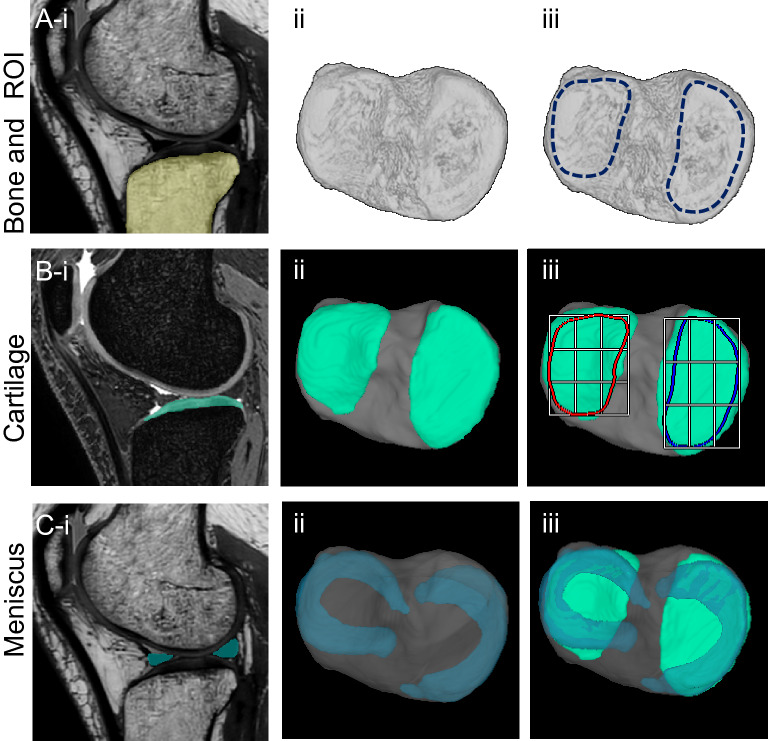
3D MRI analysis of the tibial surface of a representative control knee. (A) Bone and ROIs. The PDW images were used for automatic segmentation of the bone region (yellow) (i), the 3D image was then reconstructed (ii), and the medial and lateral tibial articular surfaces were defined as the ROIs (iii). (In this image, the ROIs were drawn manually for explanatory purposes.) (B) Cartilage. The SPGR images were used for automatic segmentation of the cartilage region (green) (i), the tibial articular cartilage and bone were reconstructed in 3D (ii), and ROIs with nine subregions were overlapped (iii). (C) Meniscus. The PDW images were used for automatic segmentation of the meniscus regions (blue) (i), the medial meniscus and lateral meniscus were reconstructed in 3D and overlapped on the bone (ii), and the cartilage was inserted (iii).
